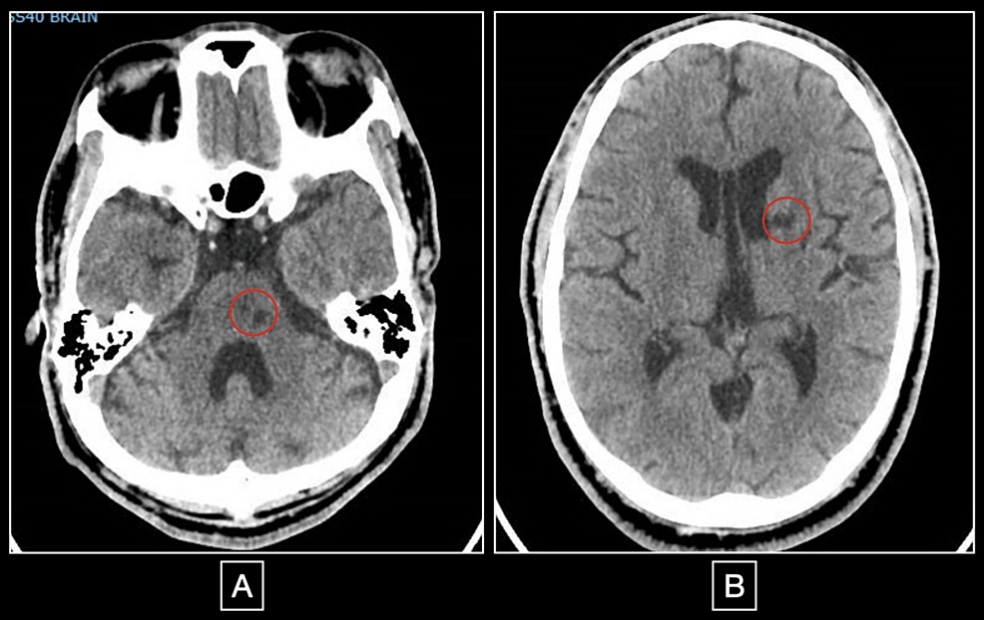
Non-contrast CT brain showing old infarcts These are 2 cuts of the non-contrast CT brain which was done in the emergency department. Figure A demonstrates a hypodensity in the left pons (red circle), which was later confirmed by MRI to be an old pontine stroke, and figure B shows a hypodensity in the left caudate nucleus (red circle) which is also consistent with an old stroke. This CT was negative for any acute pathology.
